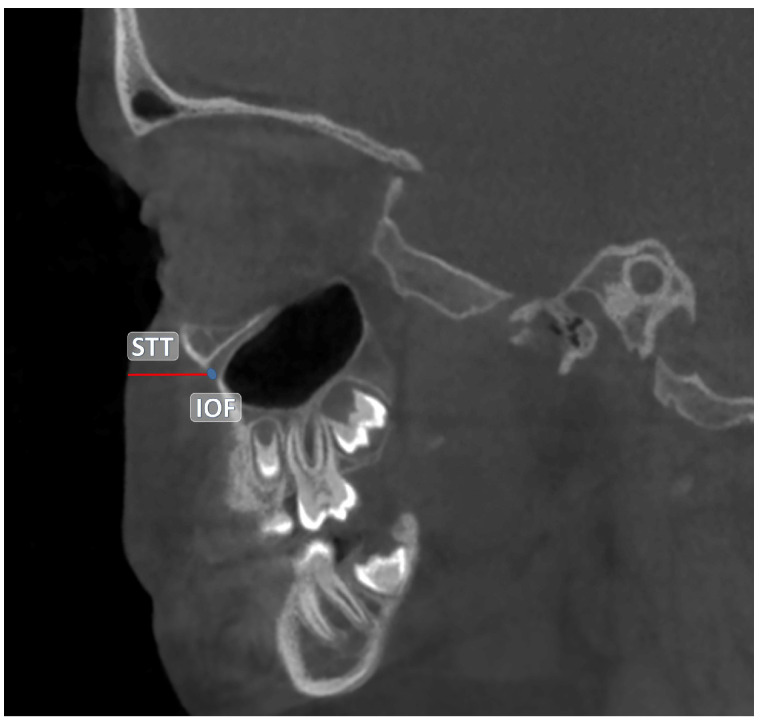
Measurement of the soft tissue thickness (STT) buccal to the IOF in sagittal CBCT sections.
